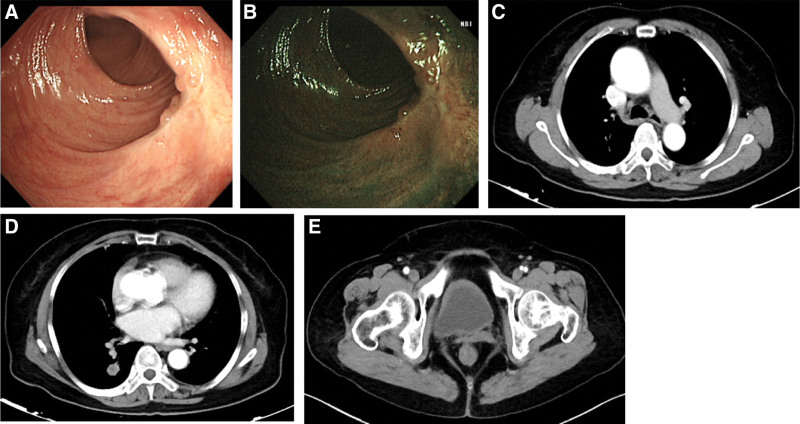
Electronic proctoscopy showed a significant reduction of rectal tumors than before treatment (A, B). CT scan revealed the neoplasms in the right upper lobe and rectal tumor shrank after treatment (C–E). CT = computed tomography.
